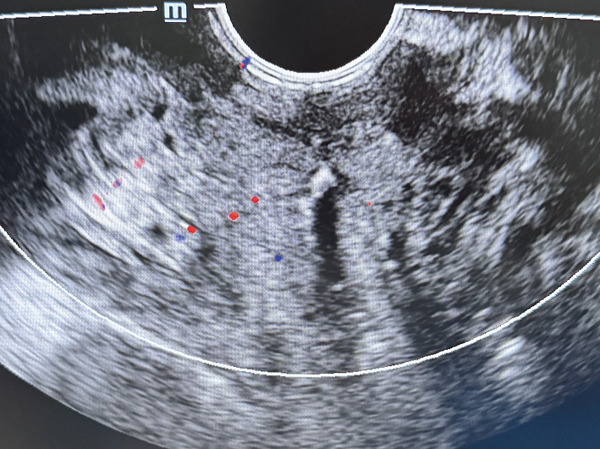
Postoperative ultrasound showing resolution of the hematoma.
